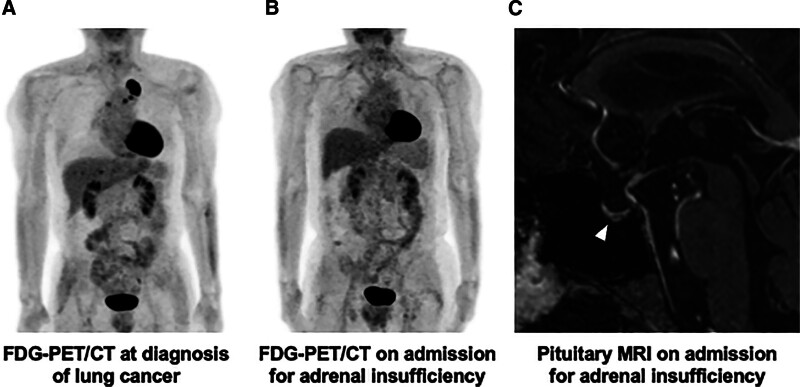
FDG-PET/CT and pituitary MRI in this subject. FDG-PET/CT at the time of diagnosis of lung cancer showed primary lung cancer in the left upper lobe, mediastinal lymph node metastasis and right adrenal metastasis (A), FDG-PET/CT during admission for suspected adrenal insufficiency showed no accumulation in the primary lung cancer, mediastinal lymph nodes, or right adrenal gland (B), and T1-weighted images of gadolinium contrast-enhanced pituitary MRI showed pituitary emptying (C). FDG-PET/CT = 18F-fluorodeoxyglucose positron emission tomography computed tomography, MRI = magnetic resonance imaging.
